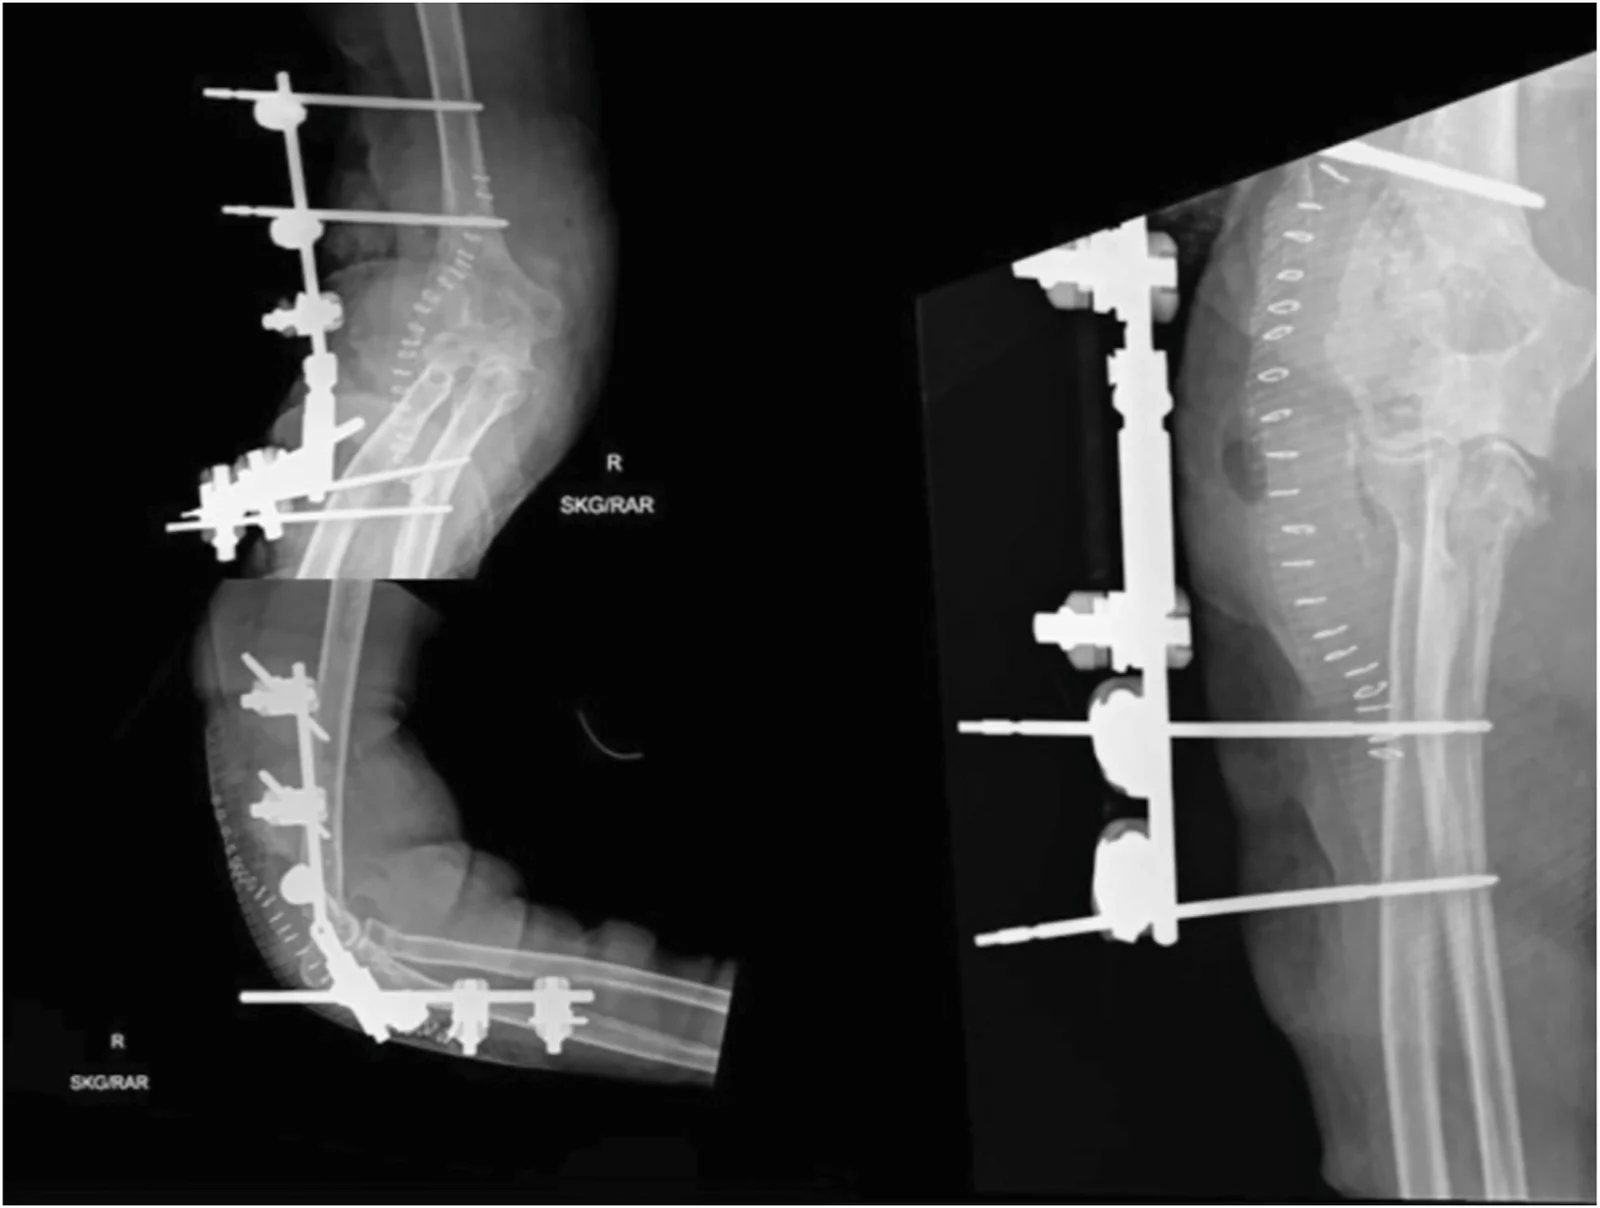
Final radiographic images of the patient's elbow post operation.
